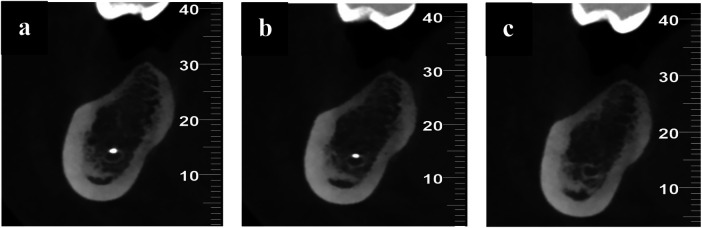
(a–c) sagittal CBCT sections taken after two years of follow-up, showing IAN canal with incomplete canal obturation.
